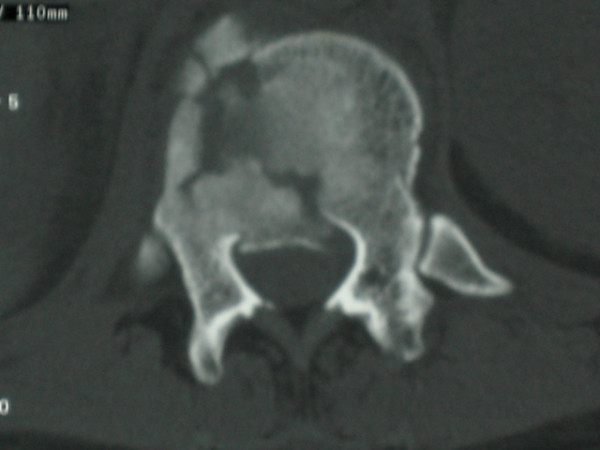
CT of spinal fracture.
